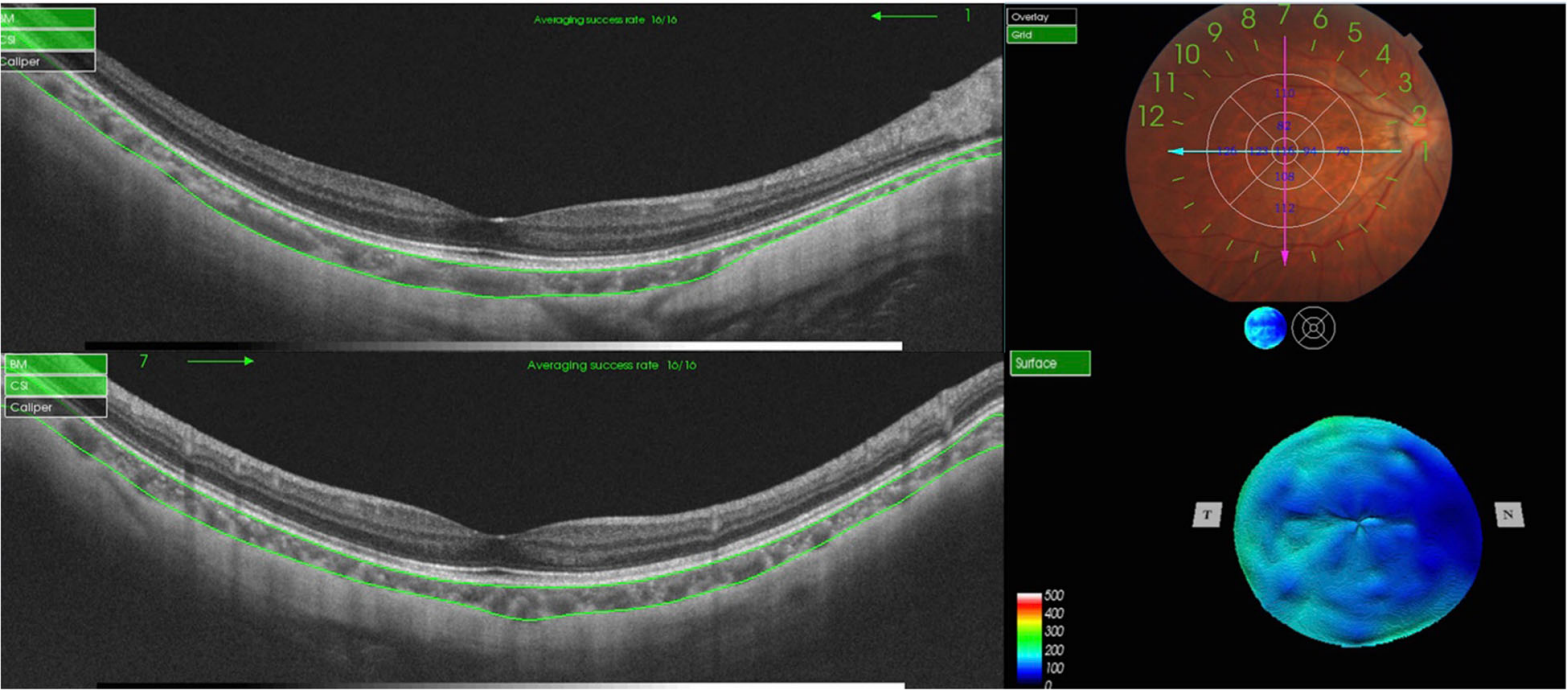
Swept-source optical coherence tomography scanning. Top and bottom left: The choroid was automatically defined as the layer between the retinal pigment epithelium and the chorioscleral interface (green line). Bottom right: CT map of the area corresponding to myopia. Top right: Study layout area and the mean regional CT calculated for the 9 sectors of the layout using the 3D macular volumetric raster scan protocol of swept-source optical coherence tomography. The units for the blue numbers are ‘μm’; BM*, Bruch’s* membrane; CSI, Chorioscleral interface; N, Nasal side; T, Temporal side.; CT, choroidal thickness
